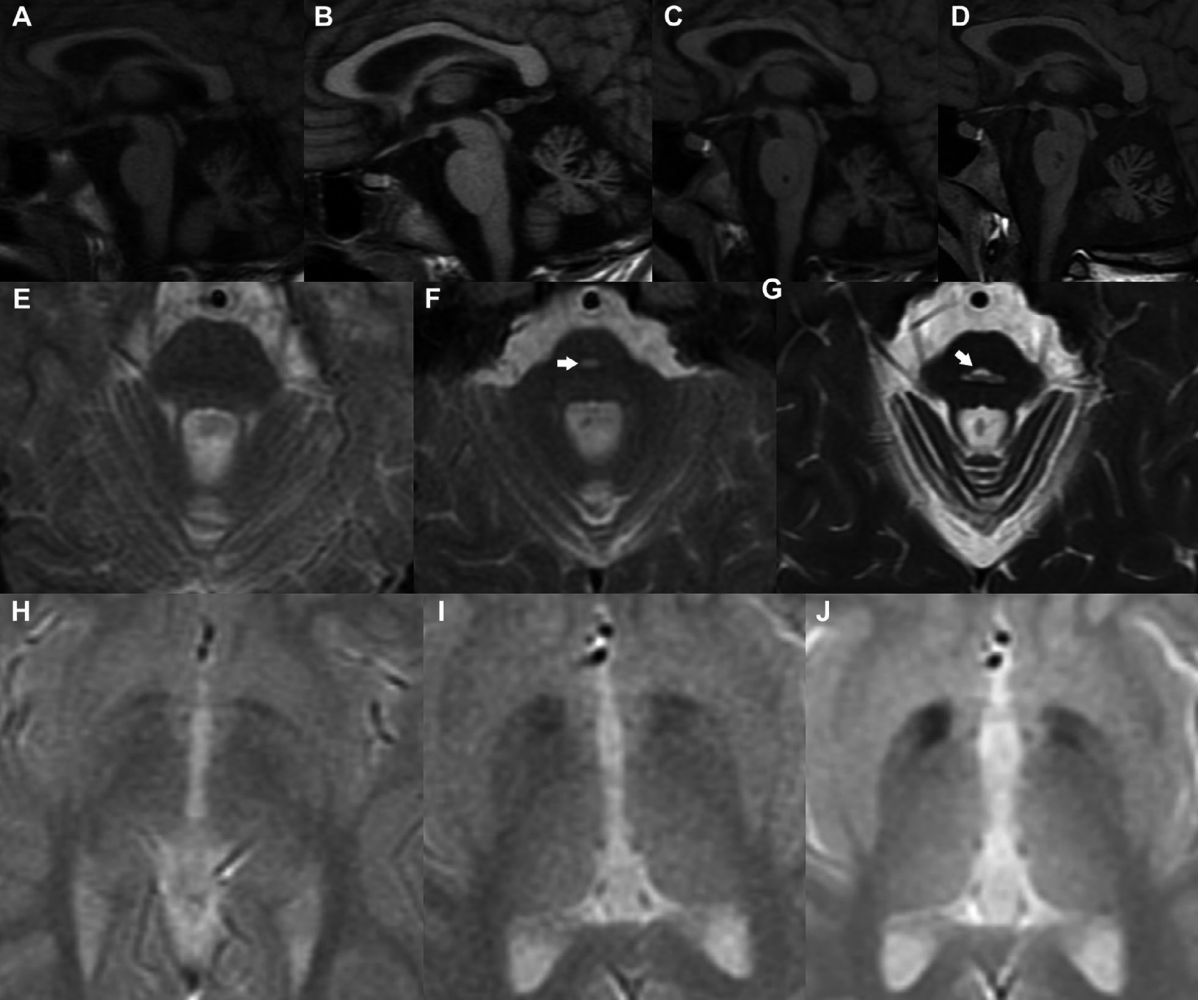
MRI proband’s studies: T1WI sagittal images (A) at 10 years old (B), 12 years old (C), 15 years old and (D) 17 years old show a progression from slight vermian atrophy to cerebellar atrophy of vermis and hemispheres, cerebral volume loss and brainstem atrophy, enlarged ventricles, and cerebral sulci at the age of 17. Axial T2WI of the pons at (E) 12 years old, (F) 15 years old and (G) 17 years old. T2 hyperintensity delineating a cross through the pons known as the “hot cross bun” sign and representing selective degeneration of pontocerebellar tracts appear at the age of 17. Gradient recall echo [GRE] sequence basal ganglia at (H) 12 years old (I), 15 years old and (J) and 17 years. Bilateral hypointensities in pallidum suggest that iron deposition appeared at 15 years.
